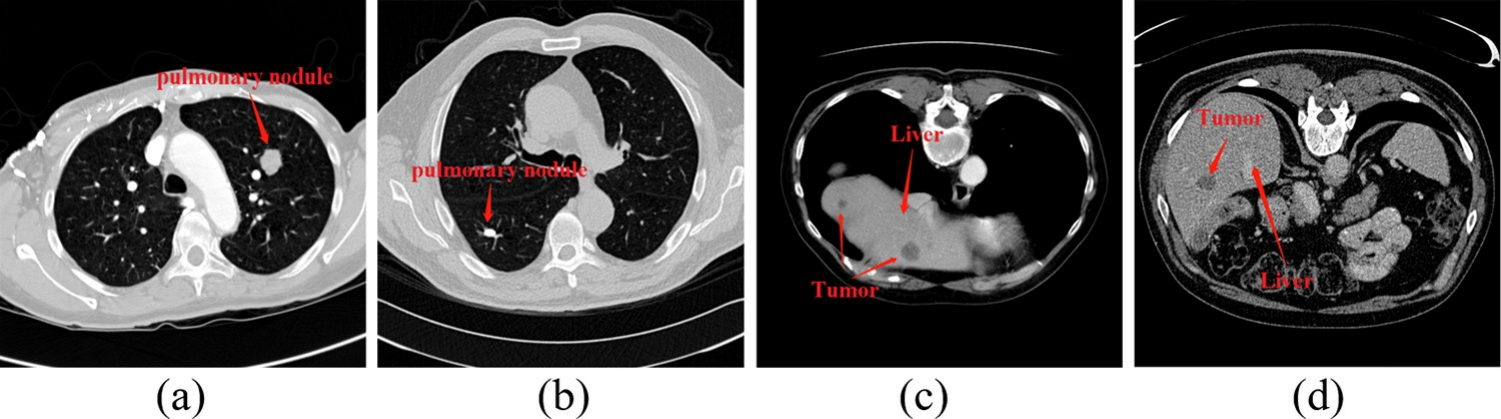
Distribution of tissue lesions in CT images. (a) and (b) are pulmonary nodules, and (c) and (d) are liver tumours.
